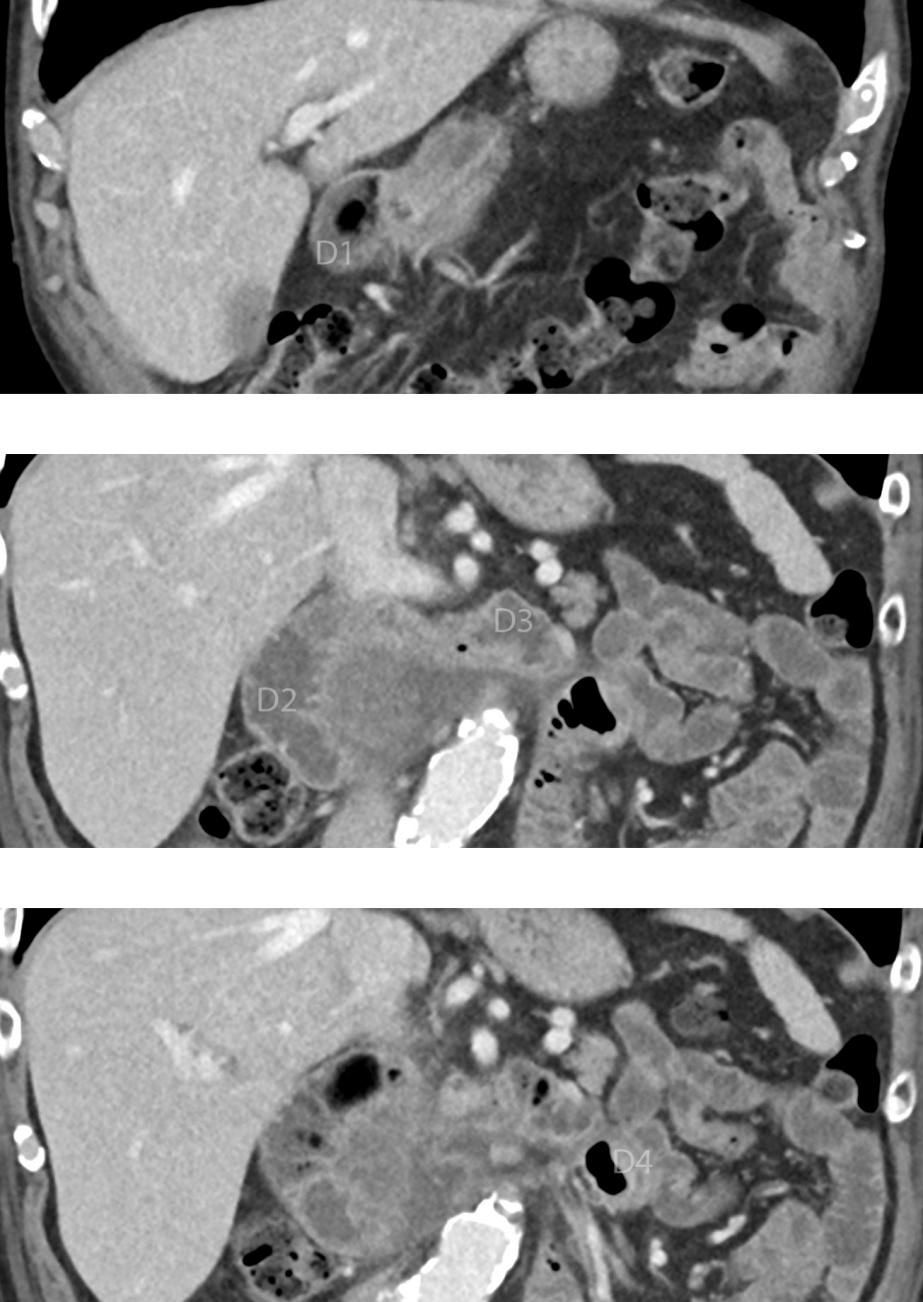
Serial CT coronal images demonstrating the D3 segment of the duodenum crossing midline in a high position in relation to D1 and the DJ flexure.
